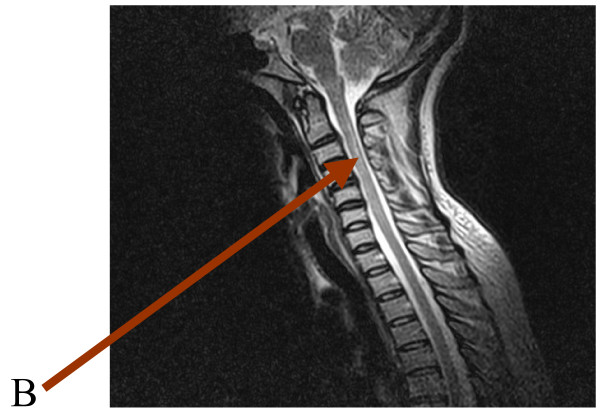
Cervical spine magnetic resonance imaging post-treatment with resolution of the previously noticed focus (Arrow B).
